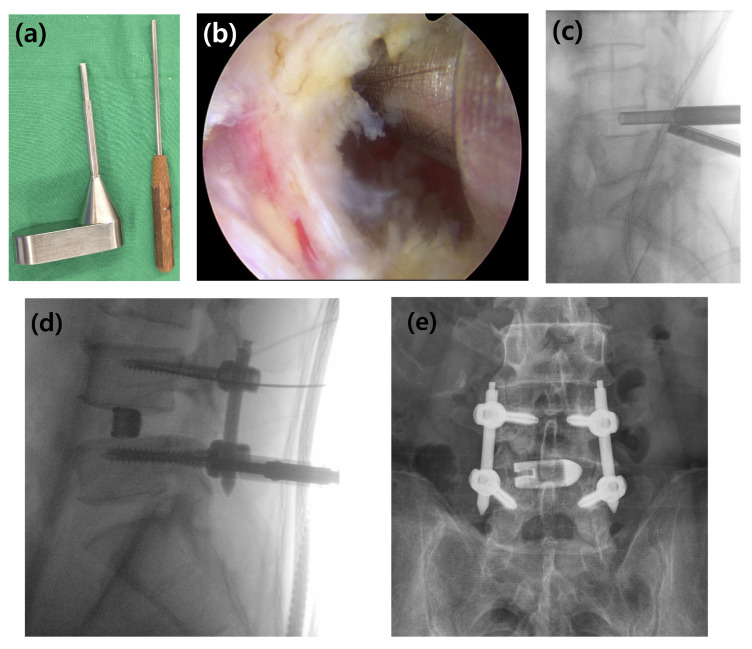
Surgical instruments and the procedure for interbody bone graft delivery. (a) The specialized bone graft funnel and the corresponding impactor used to deliver the graft material into the disc space. (b) Intraoperative endoscopic view showing the funnel correctly positioned at the entrance of the prepared disc space. (c) Lateral C-arm image confirming the position of the funnel and demonstrating the delivery of the bone graft material into the interbody space. (d) Intraoperative lateral C-arm image showing the insertion of percutaneous pedicle screws for posterior stabilization after cage placement. (e) Final anteroposterior radiograph showing the completed construct with the cage and pedicle screws.
